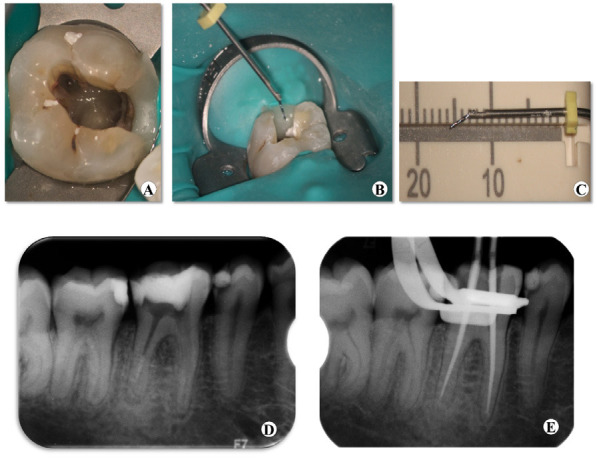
(A) After refining the access cavity and removal of intracanal medication the broken instrument could be observed in the mesiobuccal root canal under DOM. (B) Micro-tube used to retrieve the broken instrument from the mesiobuccal root canal. (C) Confirming the length of the broken instrument=3.5mm. (D) Radiograph confirming complete removal of the broken instrument. (E) Radiograph of the master cone gutta-percha.
